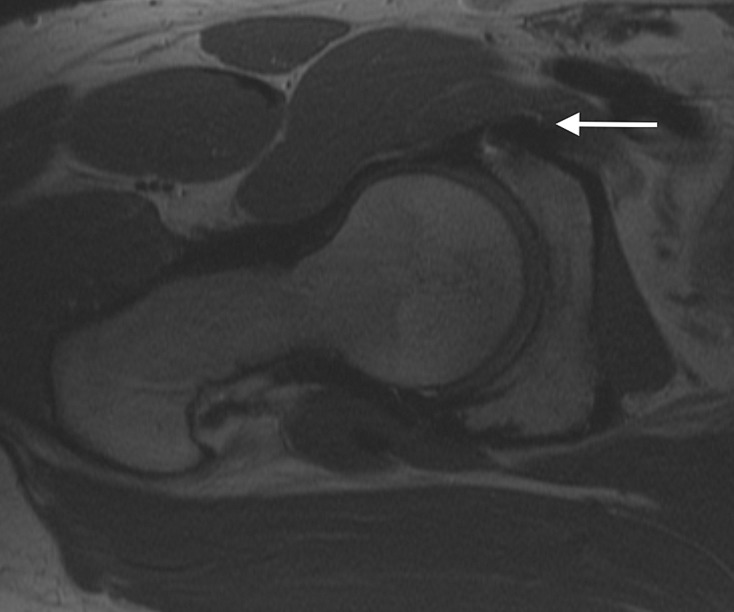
Axial MRI demonstrating medial cortical perforation with prominent suture anchor (white arrow) 77 × 64 mm.
